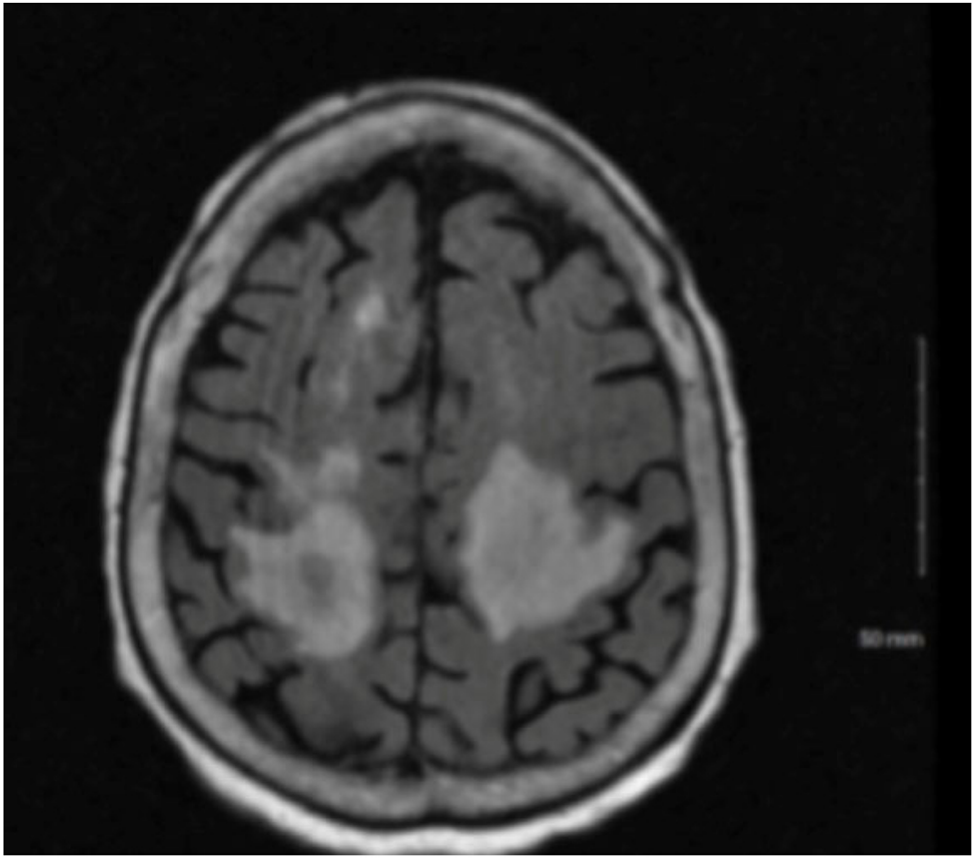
Brain MRI of an 88-year-old female with necrotizing encephalitis demonstrating bilateral frontoparietal enhancing white matter changes and surrounding vasogenic edema.
